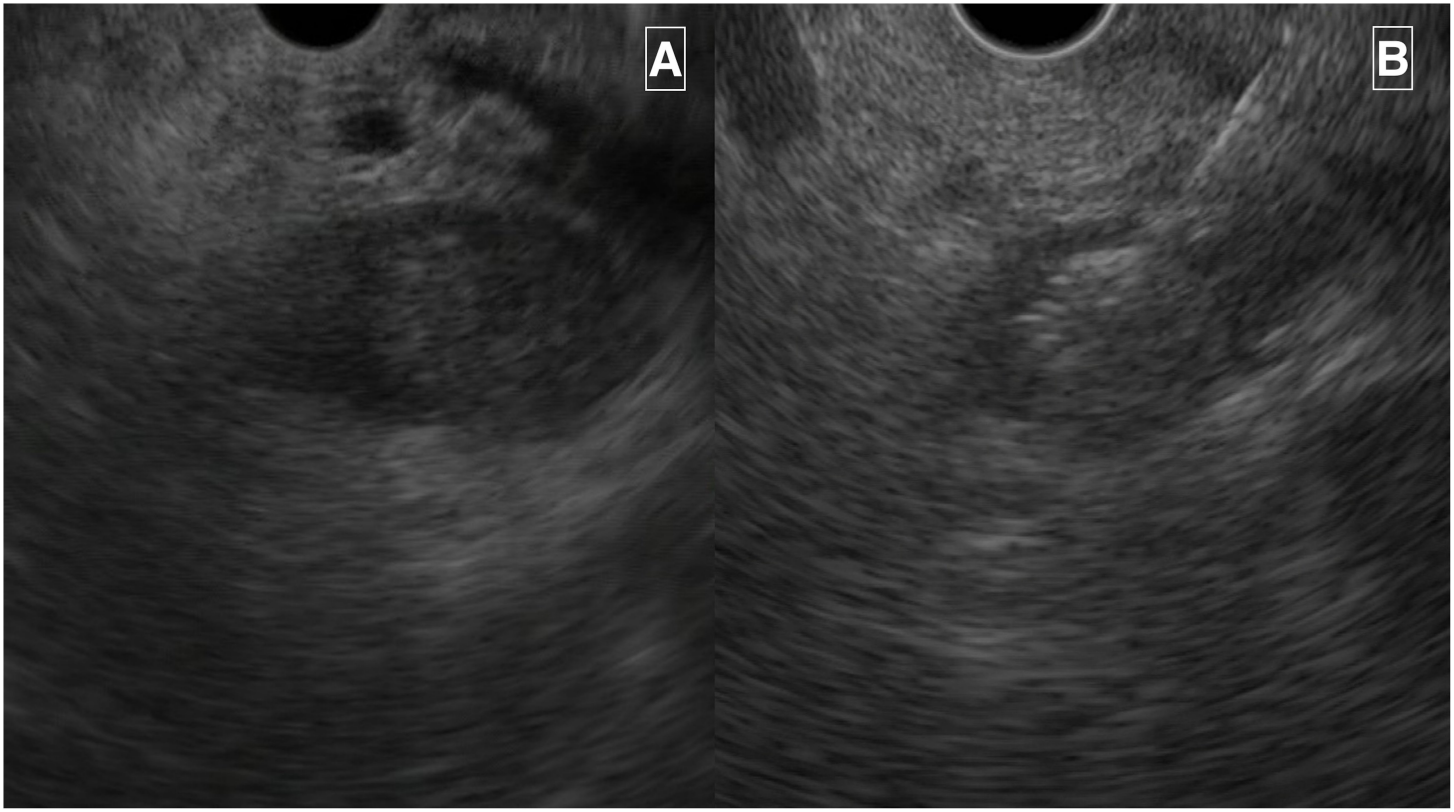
(A) Pancreatic neuroendocrine tumor visible on endoscopic ultrasound before ablation. (B) Pancreatic neuroendocrine tumor after administration of ethanol, with apparent change in its echogenicity.
